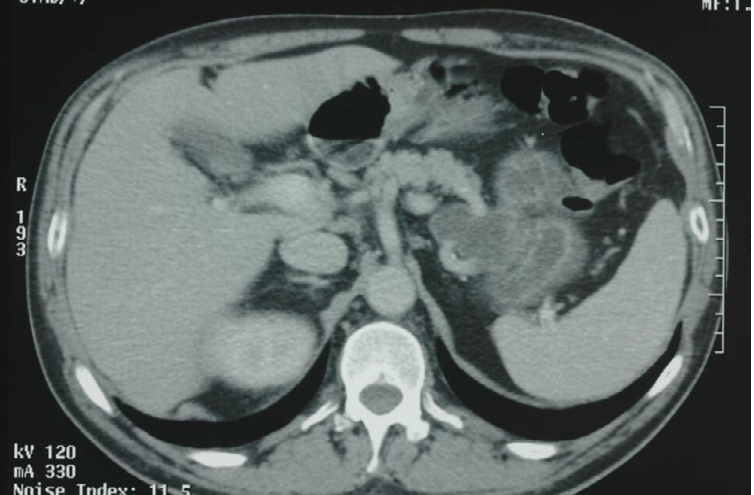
CT scan of the pancreatic cystic teratoma. Computed tomography showing a 5 cm cystic fluid mass arising from the tail of the pancreas.
